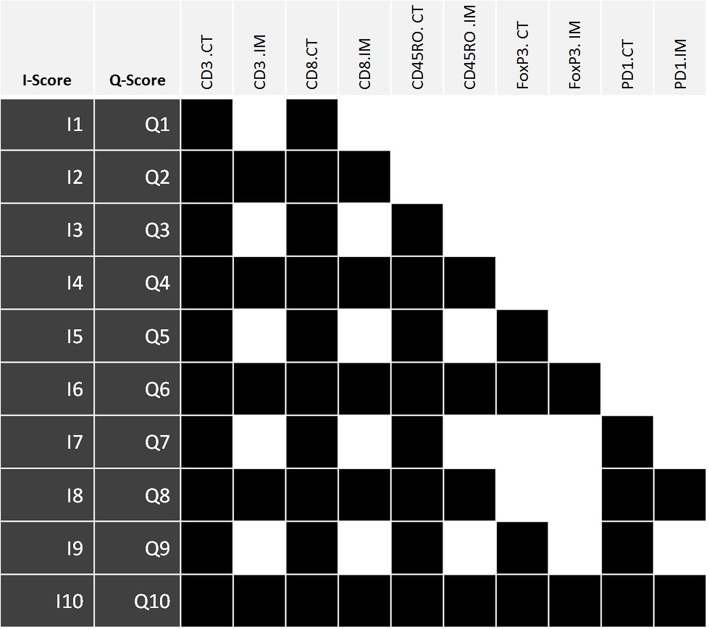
The components of immune scores I1:I10 and Q1:Q10. Each immune score is defined by the density of a set panel of immune markers (CD3, CD8, CD45RO, FoxP3, PD-1) at the tumor center (CT) +/– invasive margin (IM) relative to the corresponding densities across all tumors. The I-scores define each lymphocyte population using the median threshold methodology (0:Low, 1:High), while the Q-scores are defined using quartile ranges (0: 0–25%, 1: 25–50%, 2: 50–75%, 3: 75–100%). Therefore, for any given I-score, the corresponding Q-score presents a higher resolution scoring system. Highlighted boxes indicate inclusion of that particular lymphocyte population within an immune score. I-score, binary immune score; Q-score, quaternary immune score; CT, Tumor Center; IM, Invasive margin.
